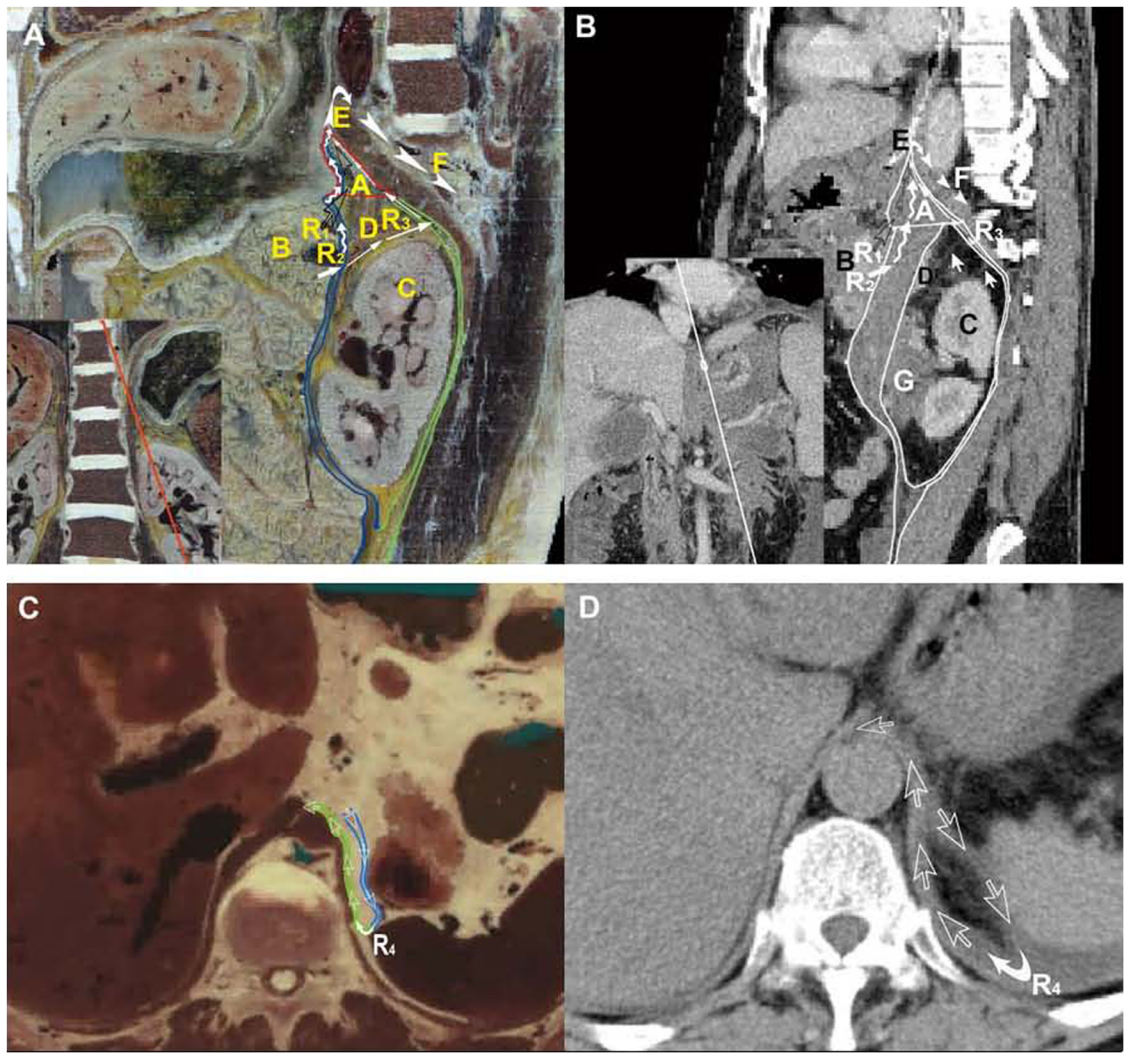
Visualization of pathways into the retrocrural space across the esophageal hiatus. (A) The oblique sagittal plane shows Route 1, Route 2 and Route 3 track together from the left extraperitoneal space to the retrocrural space across the esophageal hiatus (white arrowheads) from the lateral superior view on CVH2. Route 1 (black open arrows) is displayed as the path from the peripancreatic space to the left extraperitoneal space. Route 2 (crooked arrows) presents the conduit in the retromesenteric plane (blue lines) spreading to the left extraperitoneal space. Route 3 (white long arrows) shows the pathway from the retromesenteric plane to the retrorenal plane (green lines) and further to the left extraperitoneal space. (B) Case 1, a 47-year-old man with recurrent acute pancreatitis. Route 1 to Route 3 on the reformed CT plane corresponds well to the paths on CVH2. The thickened bridging septa distributes on Route 3 (white short arrows). A: left extraperitoneal space; B: pancreas; C: left kidney; D: left adrenal gland; E: esophageal hiatus; F: retrocrural space; G: pericapsular fluid collections. (C) Route 4 (white open arrows) from the retromesenteric plane to the left retrorenal plane across the left fascial trifurcation and further into the retrocrural space via the esophageal hiatus is displayed on the dataset of VHPA. (D) Case 2, a 60-year-old woman with recurrent acute pancreatitis. Route 4 is present in the CT image.
